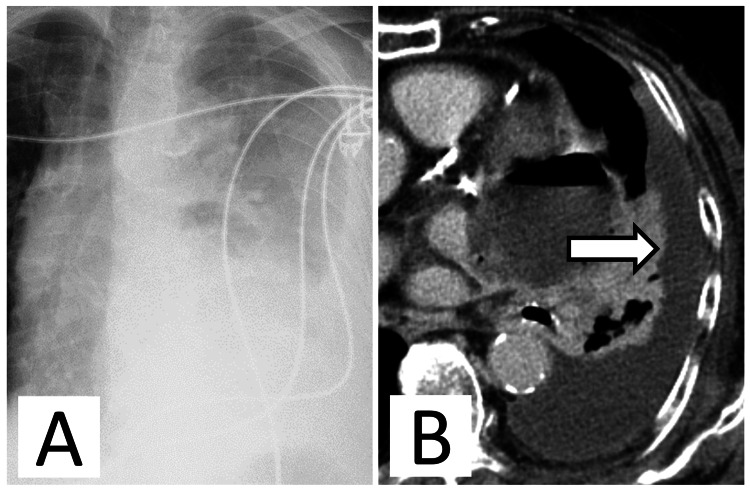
Imaging findings on the day after admission. (A) Chest x-ray showing decreased transparency in the left lung field. (B) Axial chest computed tomography revealing increased left pleural effusion (white arrow).
